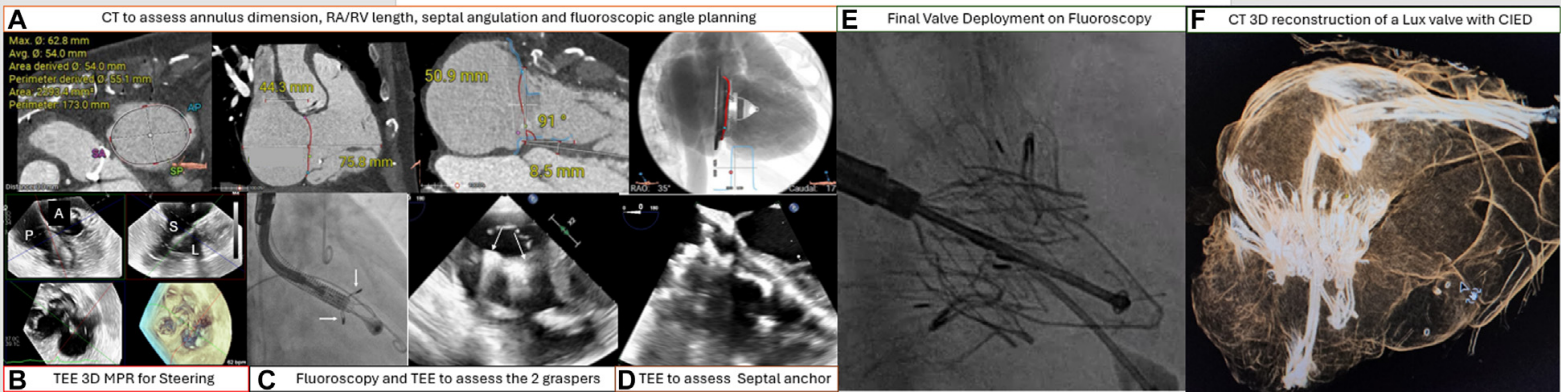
Multimodality Imaging to guide the LuX Transcatheter Tricuspid Valve Replacement (A) Preoperative computed tomography (CT) was performed to assess the tricuspid annulus size, right atrial (RA) and right ventricular (RV) length, interventricular septum angulation, and device fluoroscopic implantation projection. (B) TEE 3D MPR is used to guide the steering of delivery system. (C) Fluoroscopy and TEE STR used to assess the location of the 2 graspers (white arrows) of the LuX valve. (D) TEE at midesophageal view confirms contact of the septal anchor with the interventricular septum. (E) After septal anchor implantation, the LuX valve is deployed. (F) Follow-up imaging shows the supra-annular cuff of the LuX valve apposed to the right atrial wall to minimize any paravalvular leak, and the position of the septal anchor within the interventricular septum. Abbreviations as in Figure 5.
